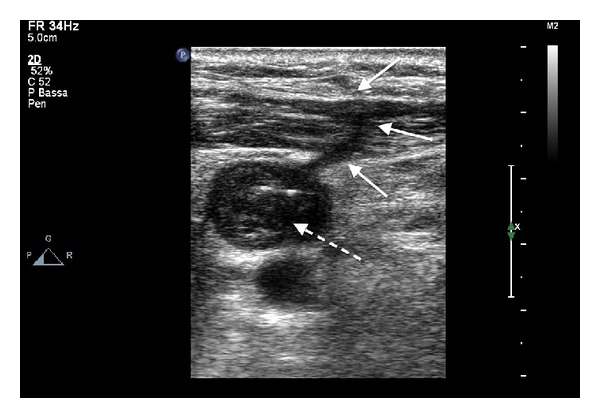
Vascular ultrasonography showing total thrombotic occlusion of the left internal jugular vein (dotted arrow) and fibrin sheath between the vein and the dermis (white arrow).
